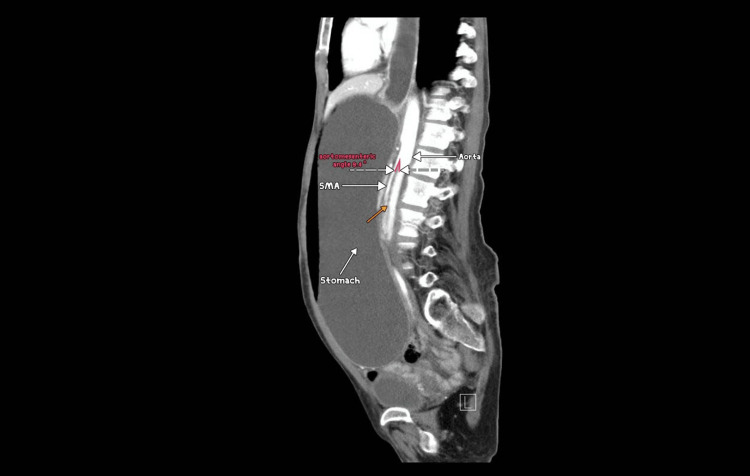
CT showing duodenum obstruction (orange arrow) between the aorta and SMA. CT: Computed tomography; SMA: Superior mesenteric artery
